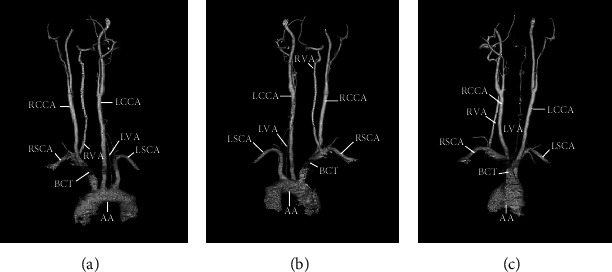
Computed tomographic angiography demonstrates the anterior-posterior (a), posterior-anterior (b), and lateral (c) views. AA = aortic arch; BCT = brachiocephalic trunk; LCCA = left common carotid artery; LSCA = left subclavian artery; RCCA = right common carotid artery; RSCA = right subclavian artery; LVA = left vertebral artery; RVA = right vertebral artery.
